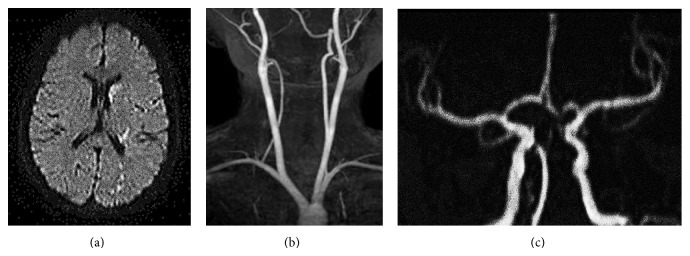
Brain MR images before cardiac tumor extraction. (a) Multiple hyperintense areas in the left basal ganglia, corona radiata, and cortex of the temporal lobe were found by diffusion-weighted MRI. (b) MR angiography did not reveal angiostenosis.
